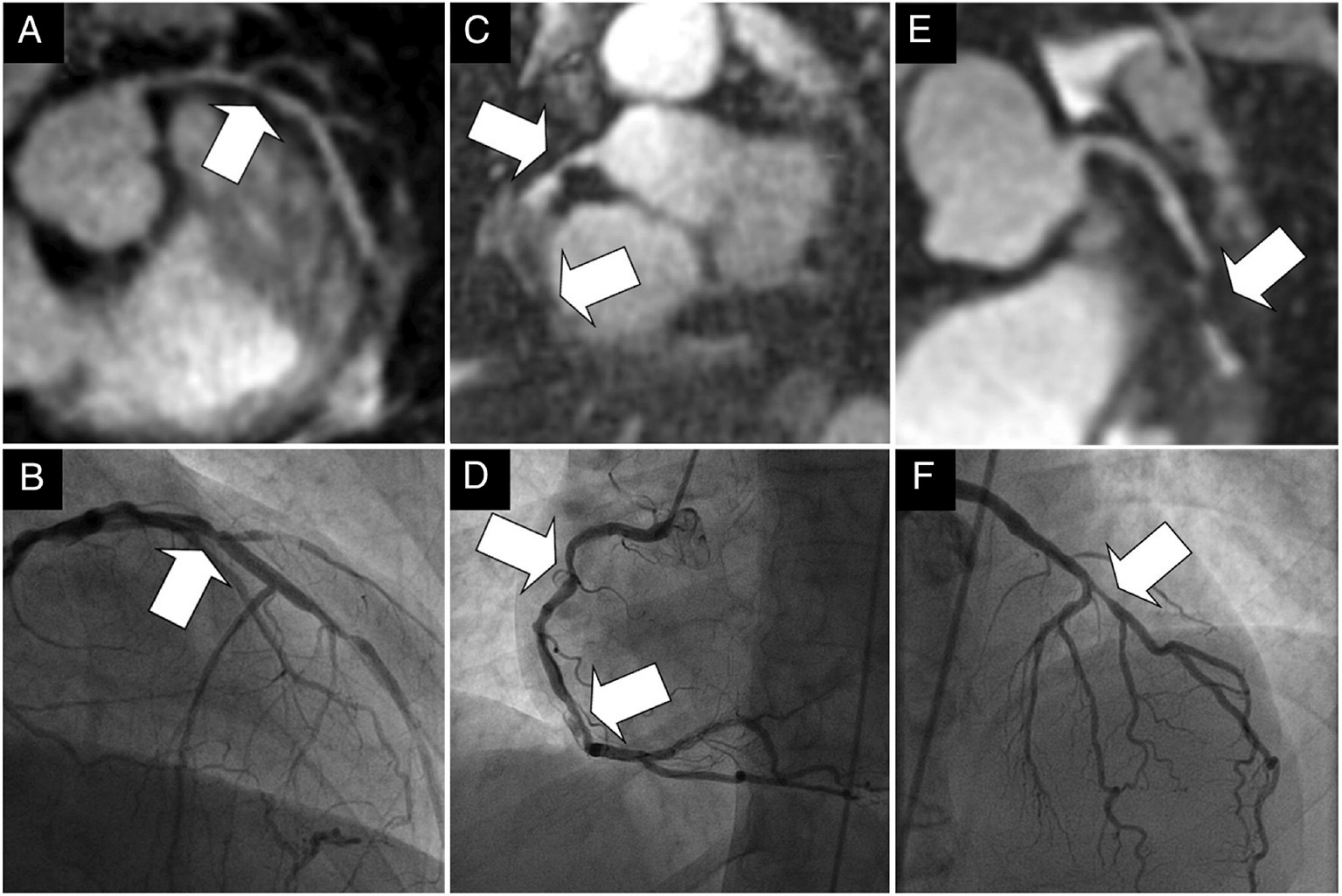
(A–F) Examples of the comparison between multiplanar reformats of the whole-heart 1D self-navigated CMRA data sets (top row) and the corresponding x-ray coronary angiograms (bottom row) in three patients. The lesion in the proximal LAD artery and just distal to the take-off of a diagonal branch can clearly be identified in the first patient in (A). while this is confirmed on the x-ray angiogram in (B). While the luminal narrowing of the proximal RCA in the second patient on (C). can clearly be identified, the further course of this artery is obscured in the region of a stent. The same in stent restenoses can be identified in (D). In the third patient in (E). significant disease is identified in the proximal LAD artery at CMRA and is confirmed on, (F). the corresponding x-ray coronary angiogram. Arrows = stenoses; LAD, left anterior descending artery; RCA, right coronary artery. Adapted with permission from Piccini et al. (46).
